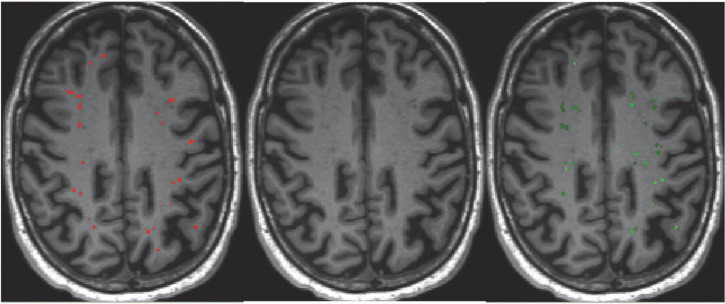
Quantification of perivascular spaces (PVS). PVS burden as shown on a T1-weighted MRI in a patient with bvFTD. (Left) Manual segmentation of PVS highlighted in red. (Right) Automated segmentation of PVS using the Multimodal Autoidentification of Perivascular Spaces (MAPS) algorithm highlighted in green.
